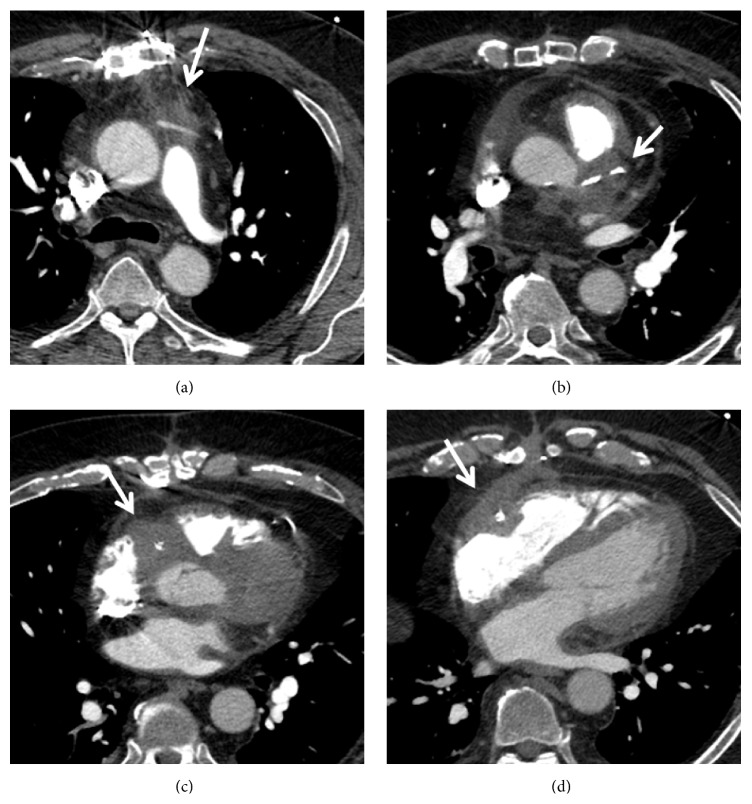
Contrast-enhanced computed tomography shows subsequent images through heart in the patient with granulomatosis with polyangiitis, extensive soft tissue attenuation around the coronary artery graft (a), left main coronary artery (b), and right coronary artery (c, d).
